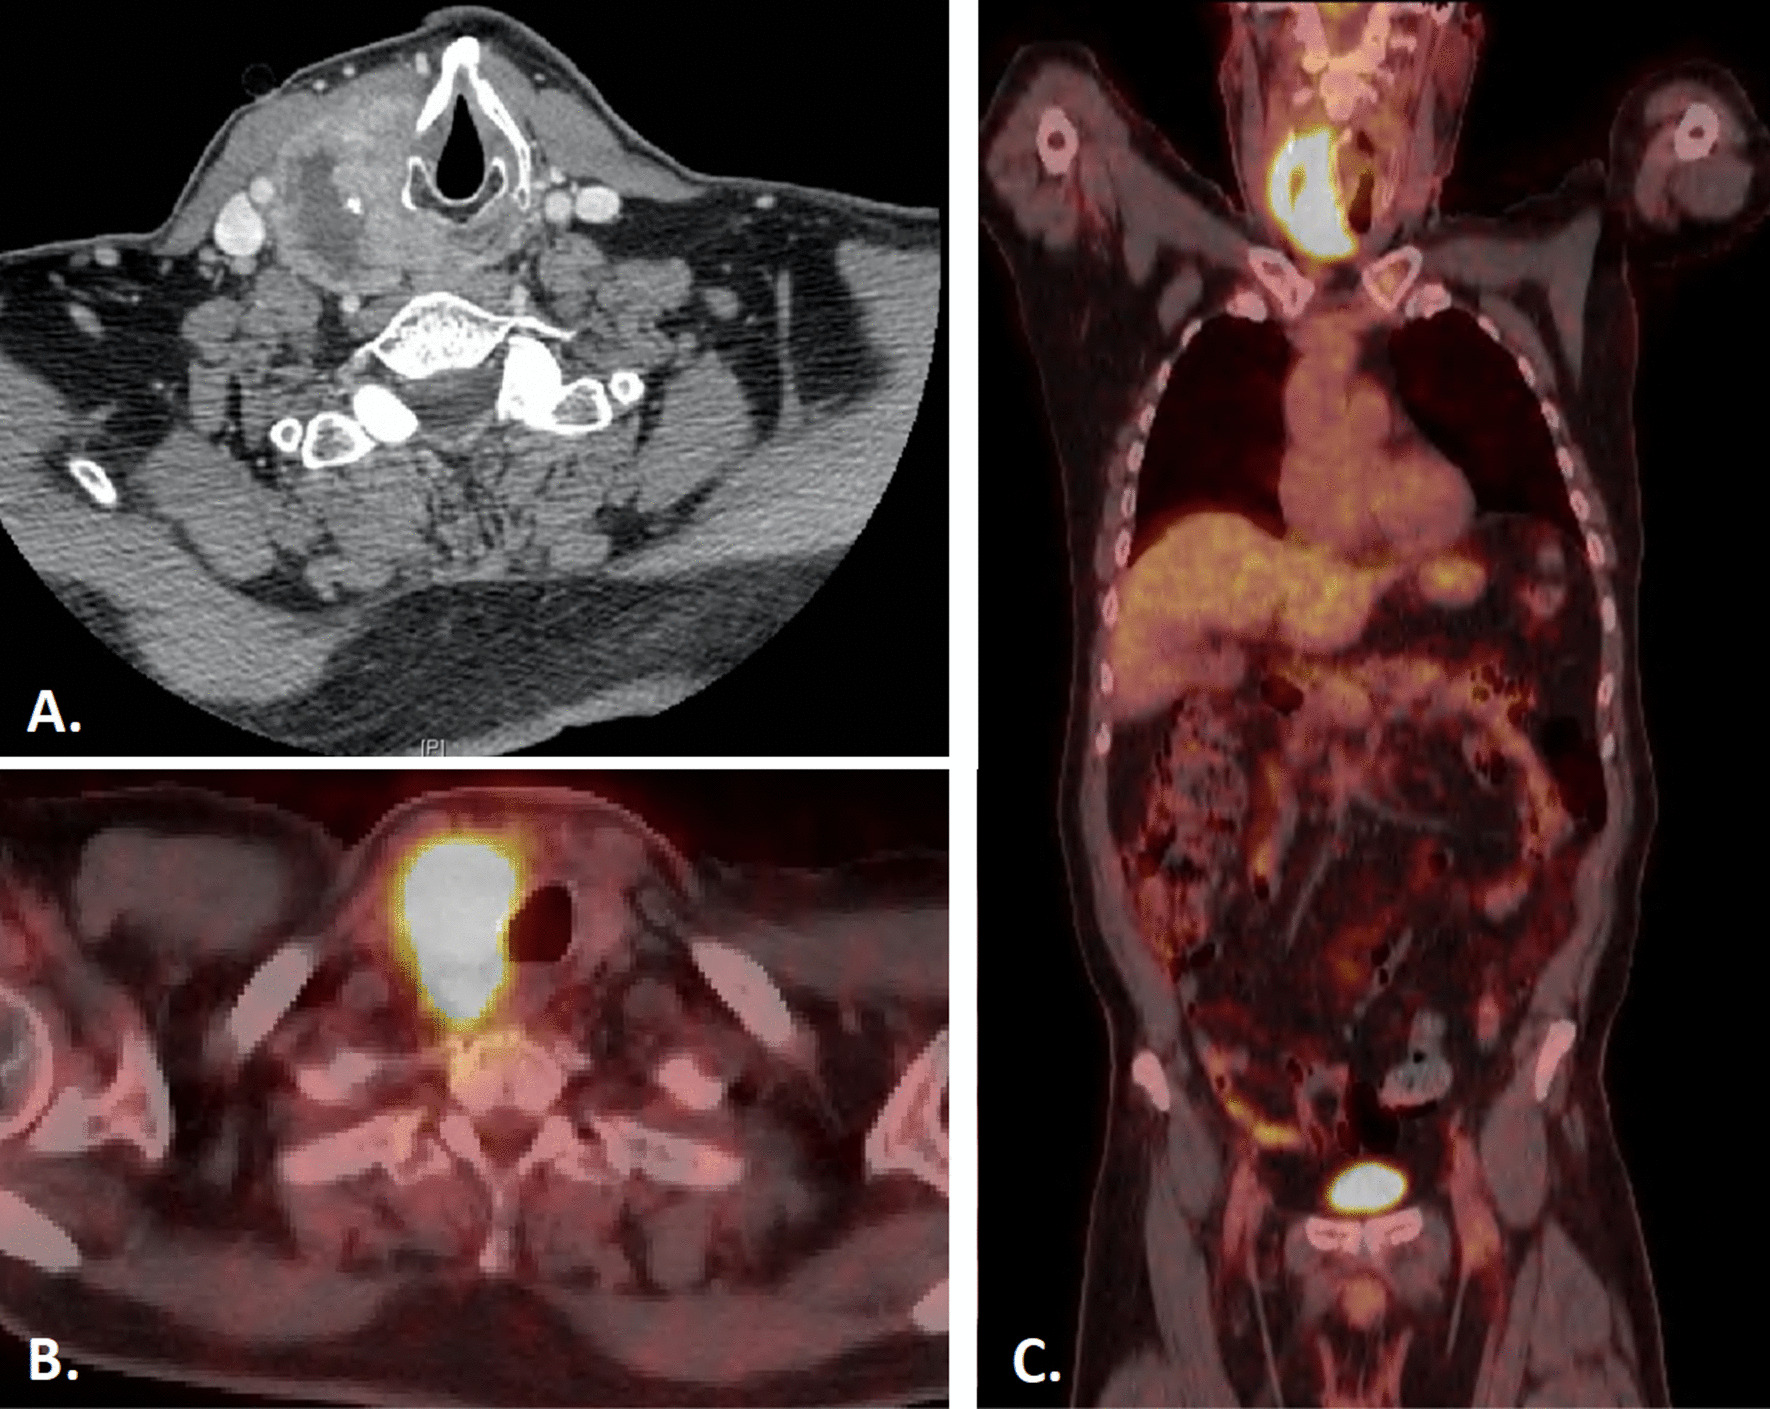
Pre-treatment diagnostic CT neck (A) and staging PET/CT (B, C) scan revealed a large right necrotic and PET-avid thyroid mass
